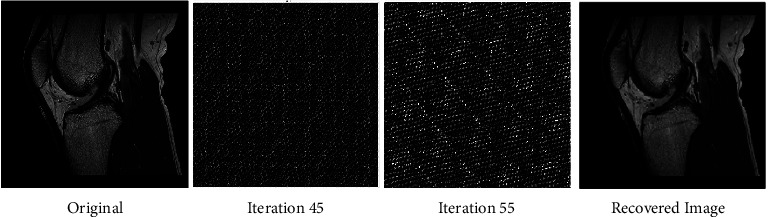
Before and after encryption of ACM (MRI knee).
